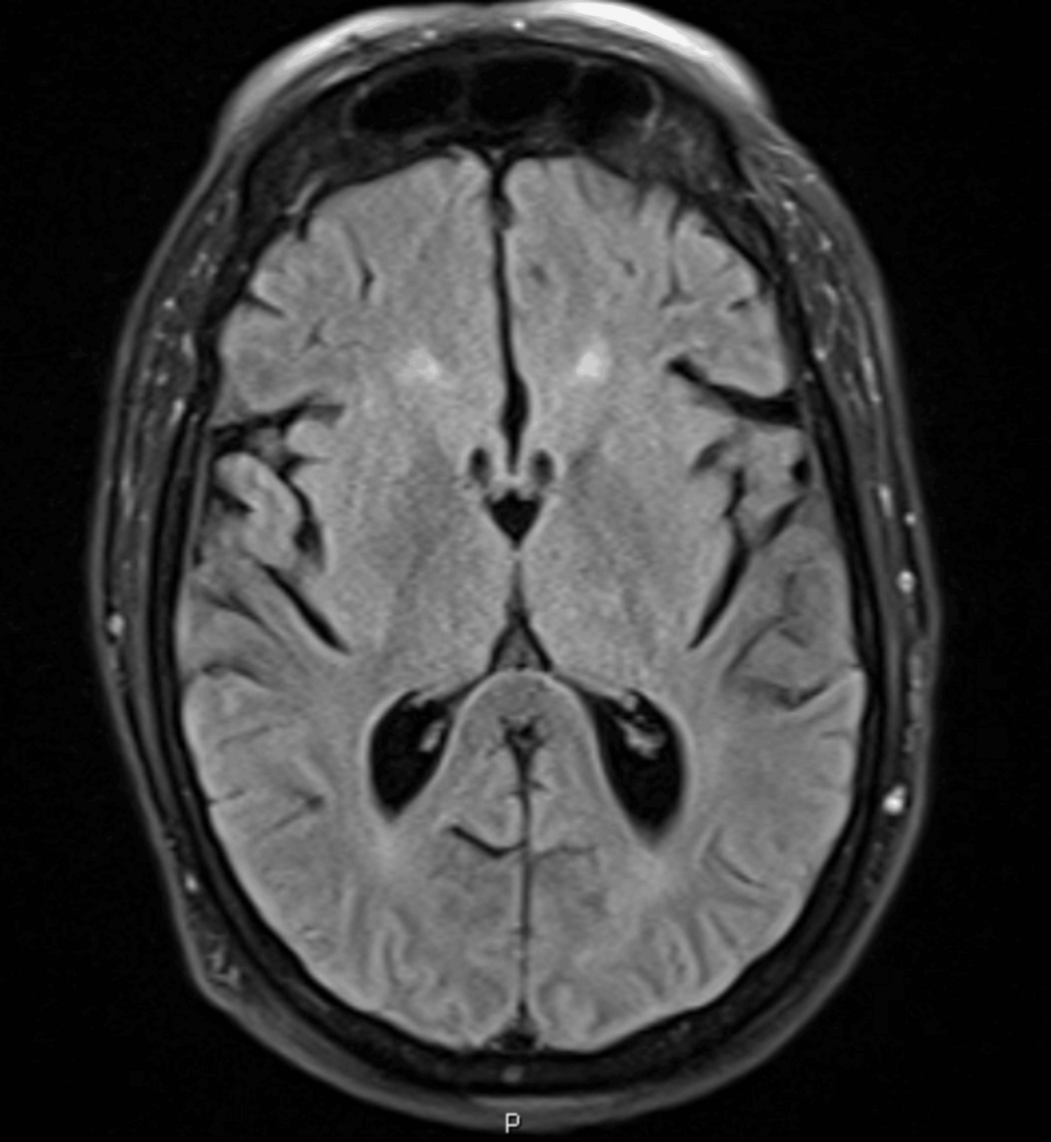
Contrast-enhanced MRI of the brain. Contrast-enhanced MRI of the brain did not reveal any parenchymal lesions or leptomeningeal enhancement.
